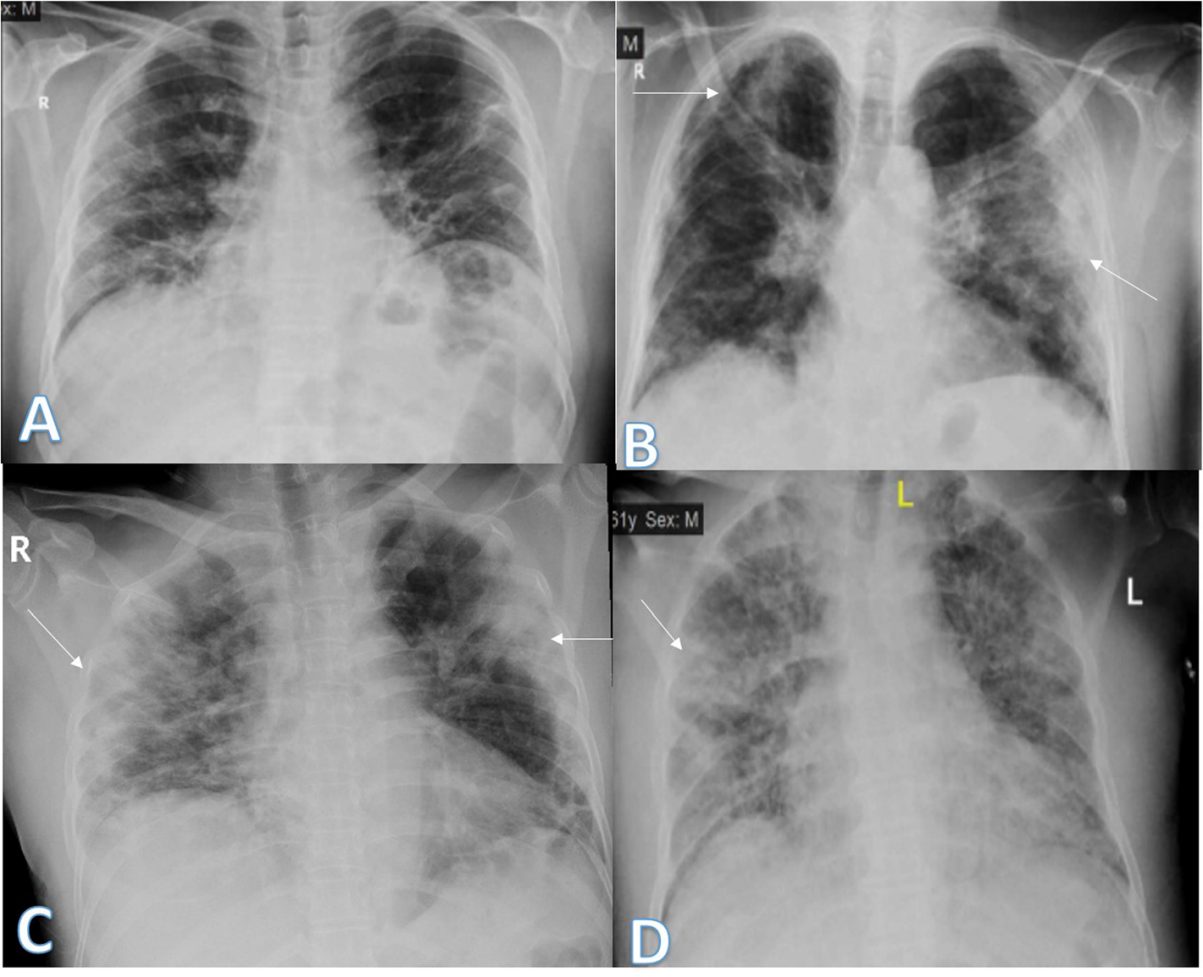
Four different patients with positive COVID-19. a 48-year male, b 48-year male, c 46-year male, and d 61-year male, follow-up chest X-ray showed bilateral diffuse reticular interstitial lung thickening with air space consolidation opacities were still seen (arrows)
